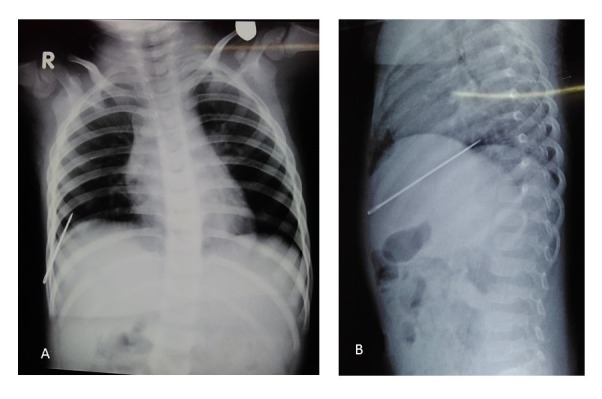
Figure 1: Chest radiograph A- PA and B-Right lateral view showing metallic foreign body in right lower chest going towards right pleural cavity.
